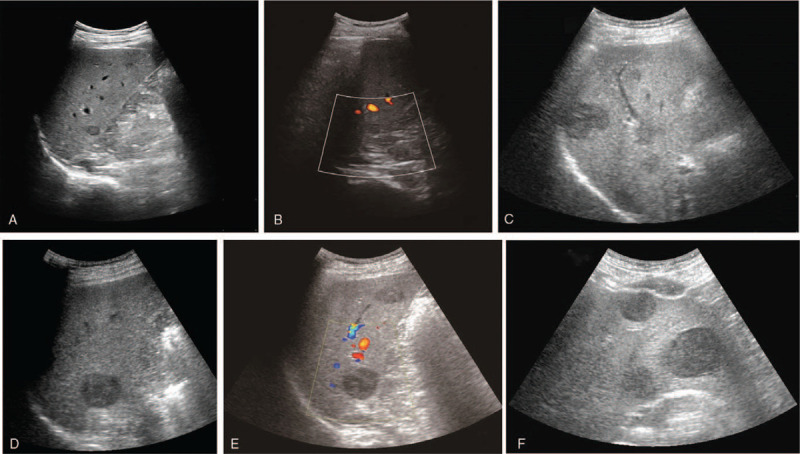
Sonographic images of nodules changing from less to more. (A) The nodule first identified by ultrasound. (B) No obvious blood flow signal was found in the nodule first identified. (D) The nodule was initially found enlarged. (E) No obvious blood flow signal was found in the nodule was initially found enlarged. (C,F) Sonographic images of a single nodule developing into multiple nodule.
